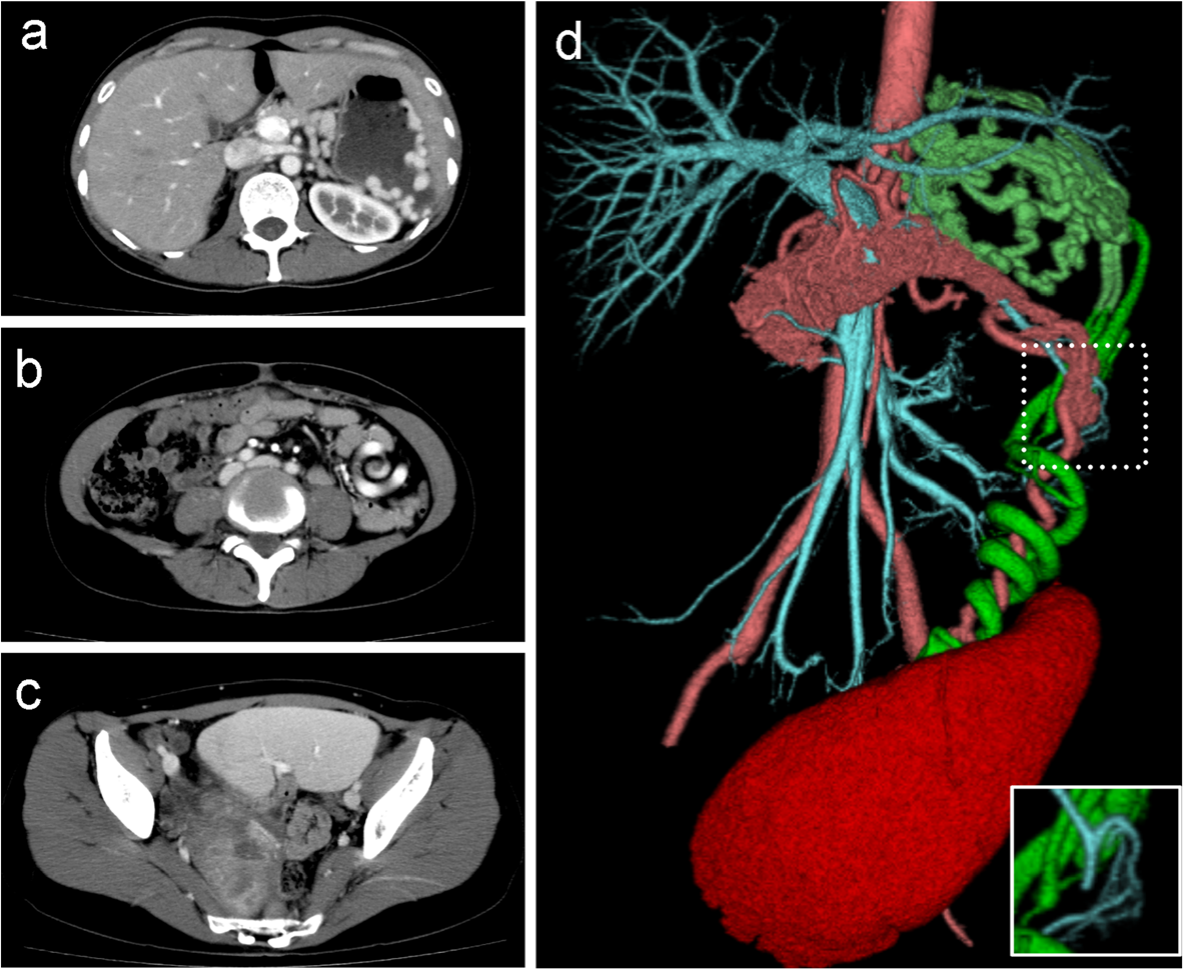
Abdominal contrast-enhanced multidetector CT imaging. (a) The isolated gastric varices on the gastric fornix. (b) The vascular pedicle showing a whirled appearance. (c) Displacement of the spleen in the lower abdomen with homogenous enhancement. (d) 3D-CT construction with vessel components. Disruption of the splenic vein at the pancreatic tail is observed in the dot square which was depicted without the arterial component in the solid square. Light blue: hepatic portal vein, splenic vein, and superior mesenteric vein. Light red: aorta, splenic artery, and small vessels in the pancreatic parenchyma. Green: collateral veins. Red: spleen.
